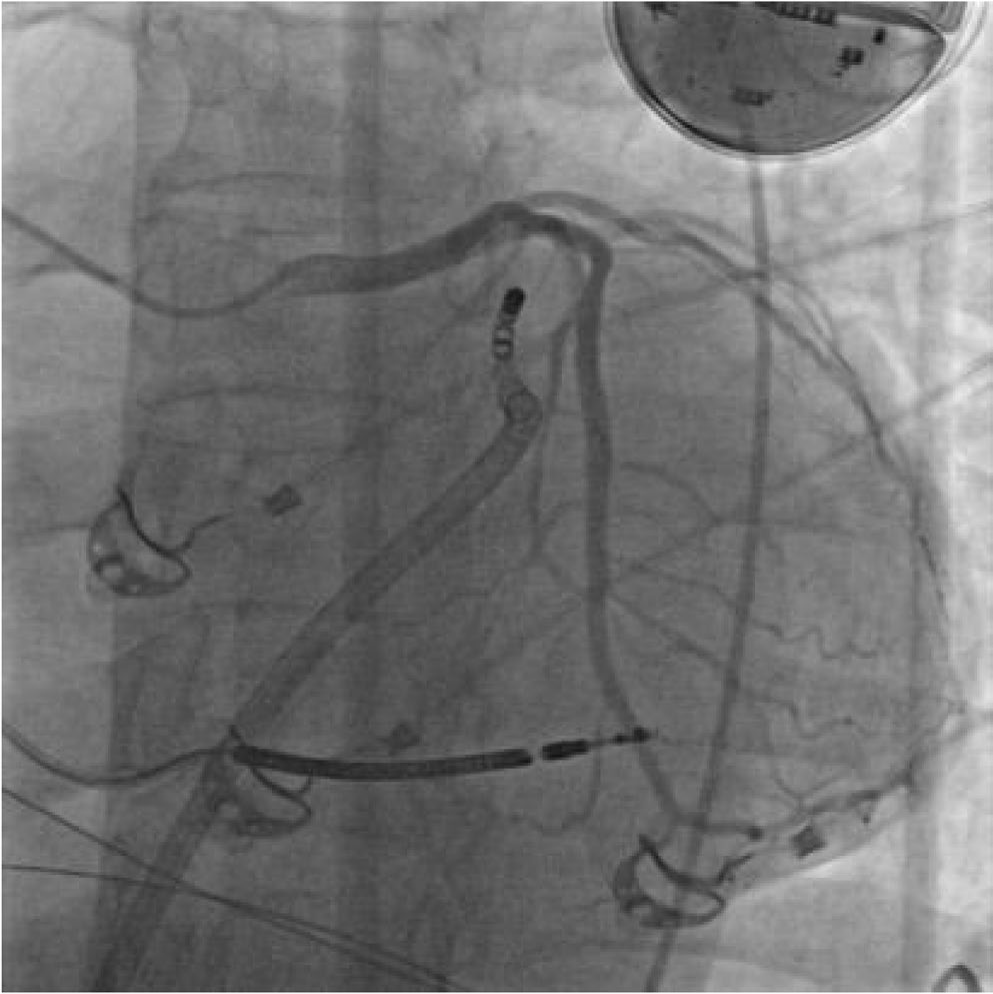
## COMPUTED TOMOGRAPHY‐VERIFIED PACING SITES OF LEADLESS MICRA™ PACEMAKERS IMPLANTED IN BRADYCARDIA PATIENTS
